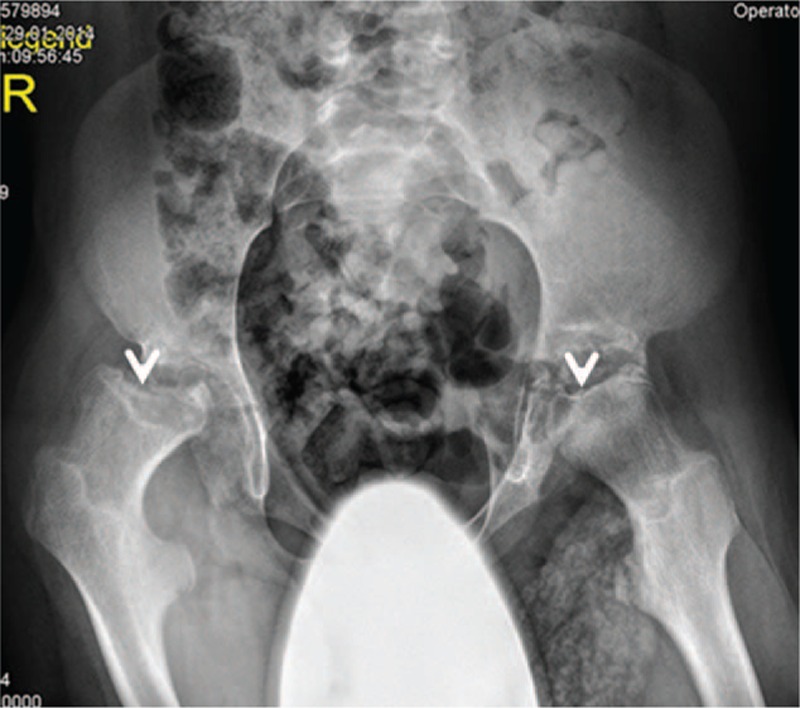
Anterior posterior pelvis radiograph, subject (IV, 10) showed femoral necks in valgus position, hypoplastic iliac bodies, and collapse of the capital femoral epiphyses (arrows) associated with unilateral dislocation of the right hip.
